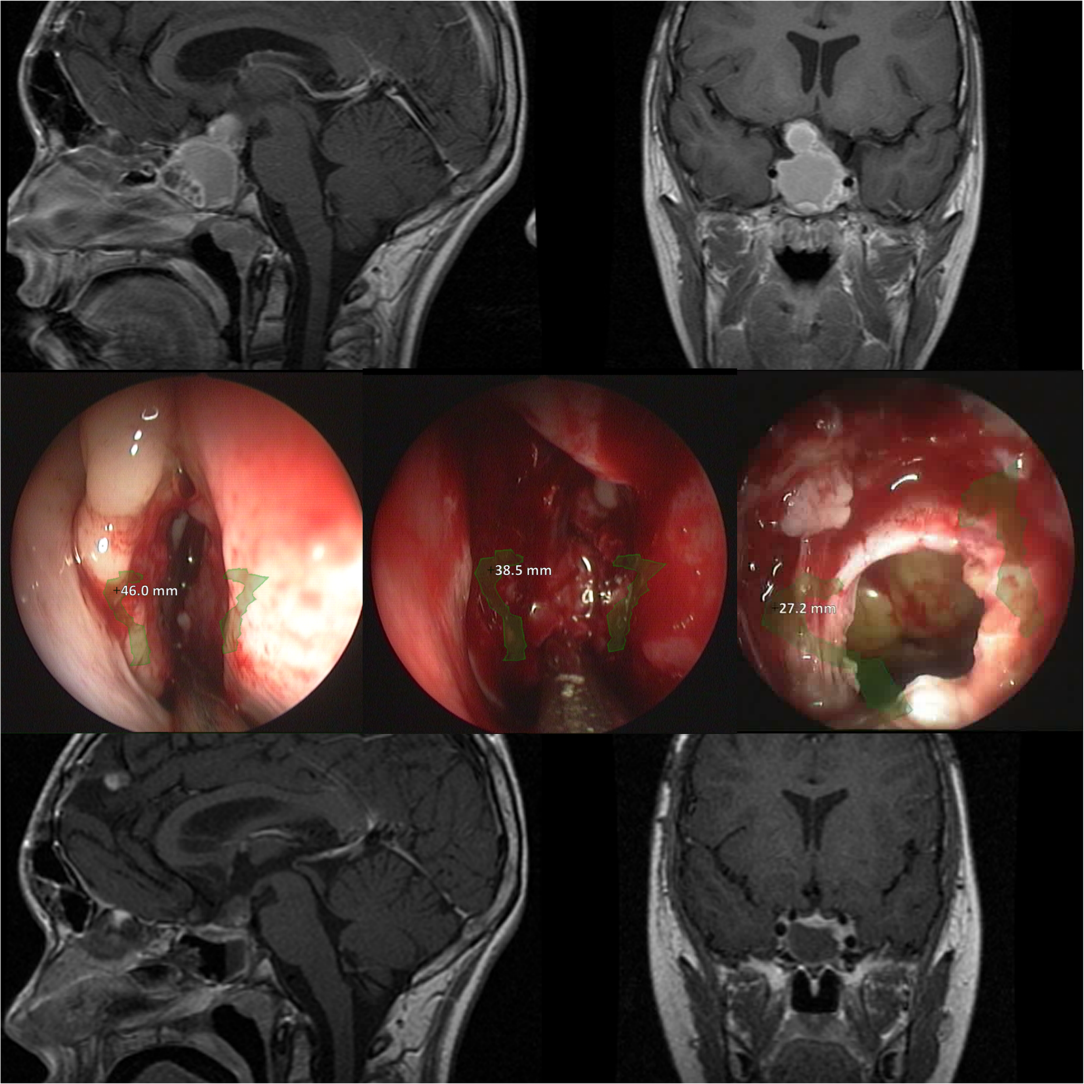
Case example of a patient with a craniopharyngioma: Upper row: preoperative MRI (T1 sagittal and coronal post-gadolinium sequences). Middle row: intraoperative endoscopic view at different anatomic levels of the turbinates, the sphenoid cavity and the sella with AR information indicating the carotid arteries. Lower row: postoperative MRI (T1 sagittal and coronal post-gadolinium sequences)
